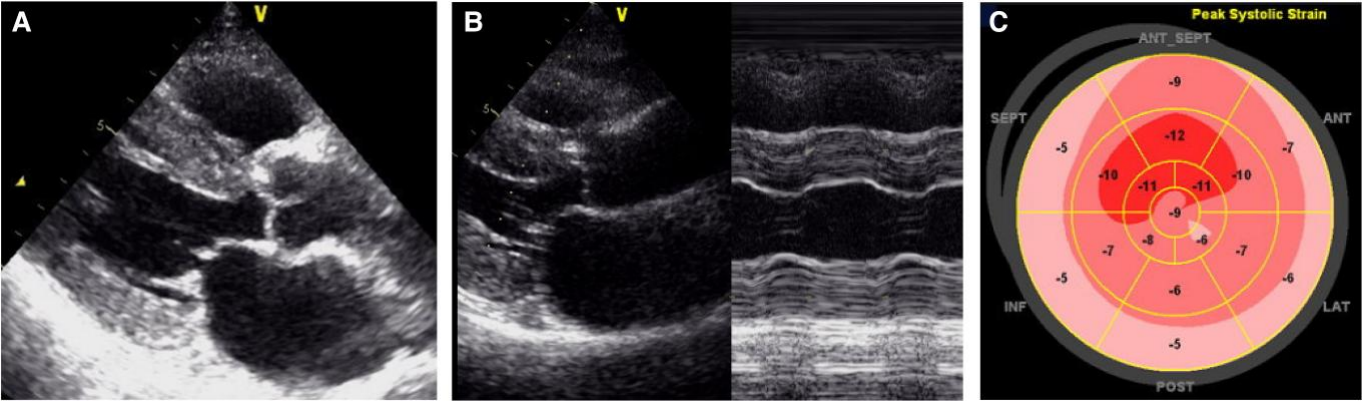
Echocardiographic findings demonstrating diffuse thickening of the left ventricular wall. Measurements: interventricular septum thickness, 12.3 mm; posterior wall thickness, 12.4 mm; left ventricular end-diastolic diameter, 37 mm; left ventricular end-systolic diameter, 27 mm; and left ventricular ejection fraction, 57%. (*A*) Parasternal long-axis view. (*B*) M-mode imaging. (*C*) Left ventricular longitudinal strain.
